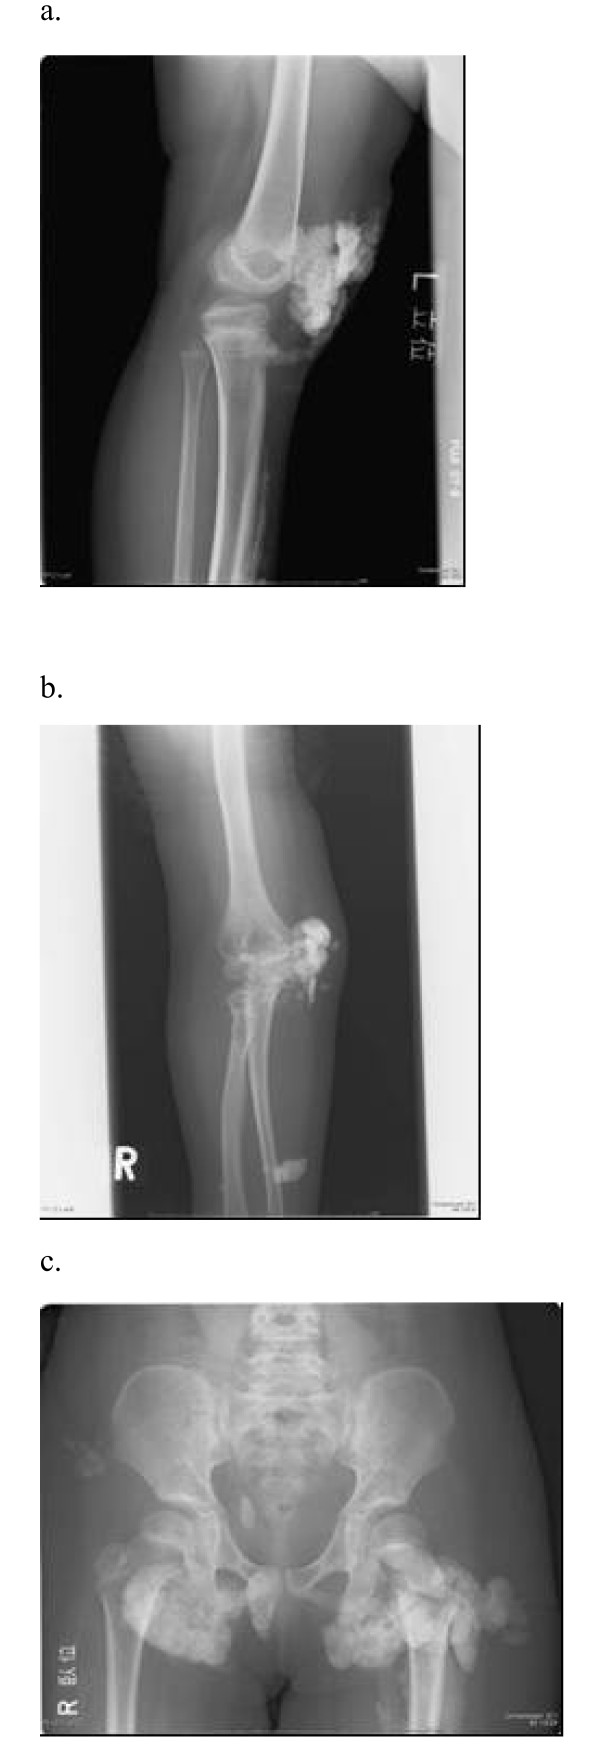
X-ray findings of extremities and pelvis. a. Calcium deposit around right knee in April 2001, 3 years and 3 months after JDM onset. b. Subcutaneous calcium nodule over right elbow in January 2003, 5 years after JDM onset. c. Tumoral calcinosis in the buttocks in September 2003, 5 years and 8 months after JDM onset.
